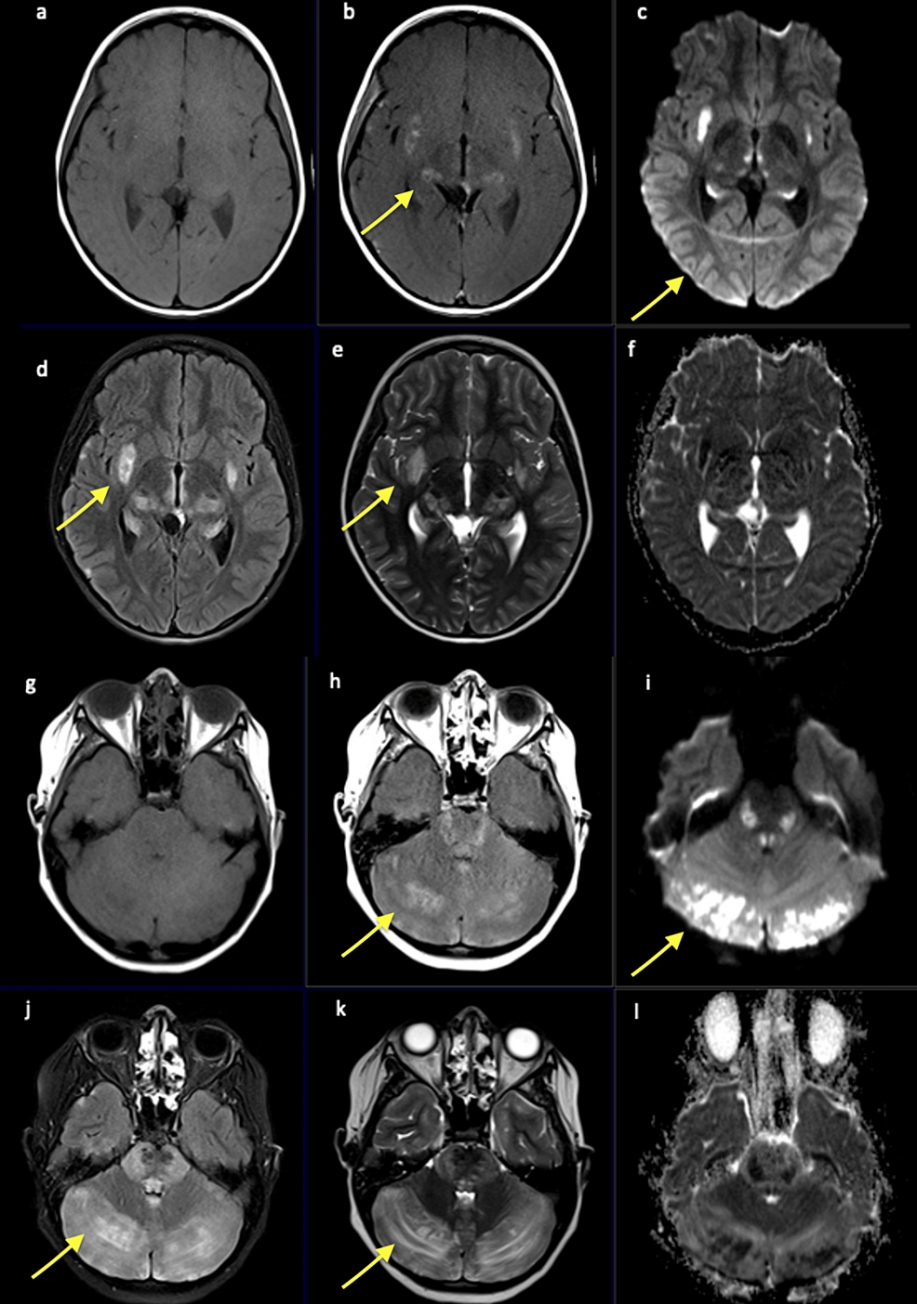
Axial T1-weighted imaging (a-g) and gadolinium-enhanced T1- weighted imaging (b-h), diffusion-weighted imaging (c-i), axial FLAIR-weighted imaging (d-j), T2-weighted imaging (e-k), and apparent diffusion coefficient (f-l) demonstrating multifocal areas of T2/FLAIR hyperintensity (indicated by yellow arrows) with areas diffusion restriction involving the bilateral right greater than left external capsule/lateral putamen, bilateral medial thalami, and amygdala, hippocampus, hypothalamus, midbrain, pons, superior cerebellar peduncle, and cerebellum, vermis, and brainstem. FLAIR: Fluid-attenuated inversion recovery
